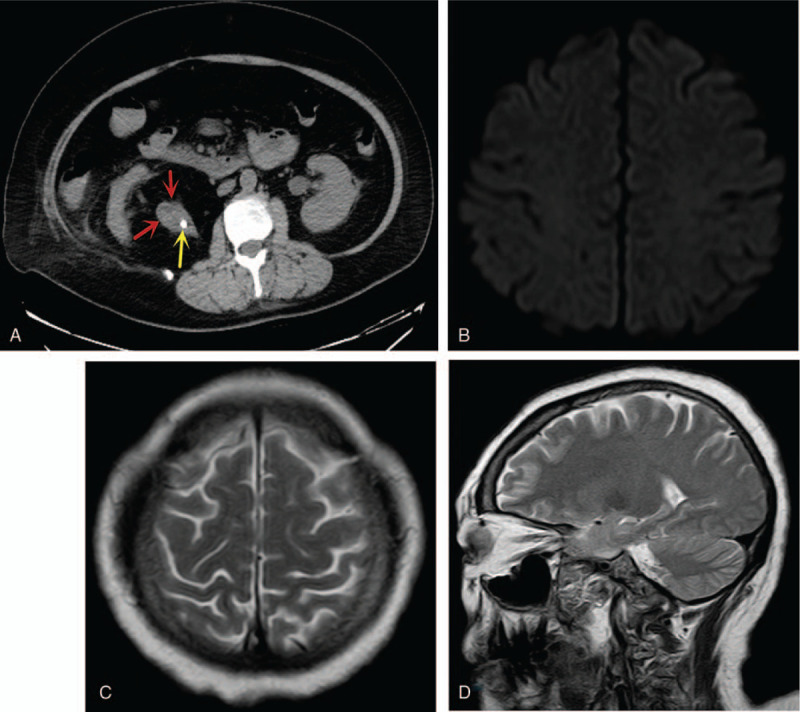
(A) Is a CT scan showing a total descend of the right ureteric stone into the bladder and a resolution of the dilatation of right ureter. Red arrow = ureter, yellow arrows = stone. (B–D) Are repeated MRIs of the head showing disappearance of the abnormal signals at both TPO cortices, the subcortical area, as well as the left hippocampus. CT = computer tomography, TPO = temporo-parieto-occipital.
